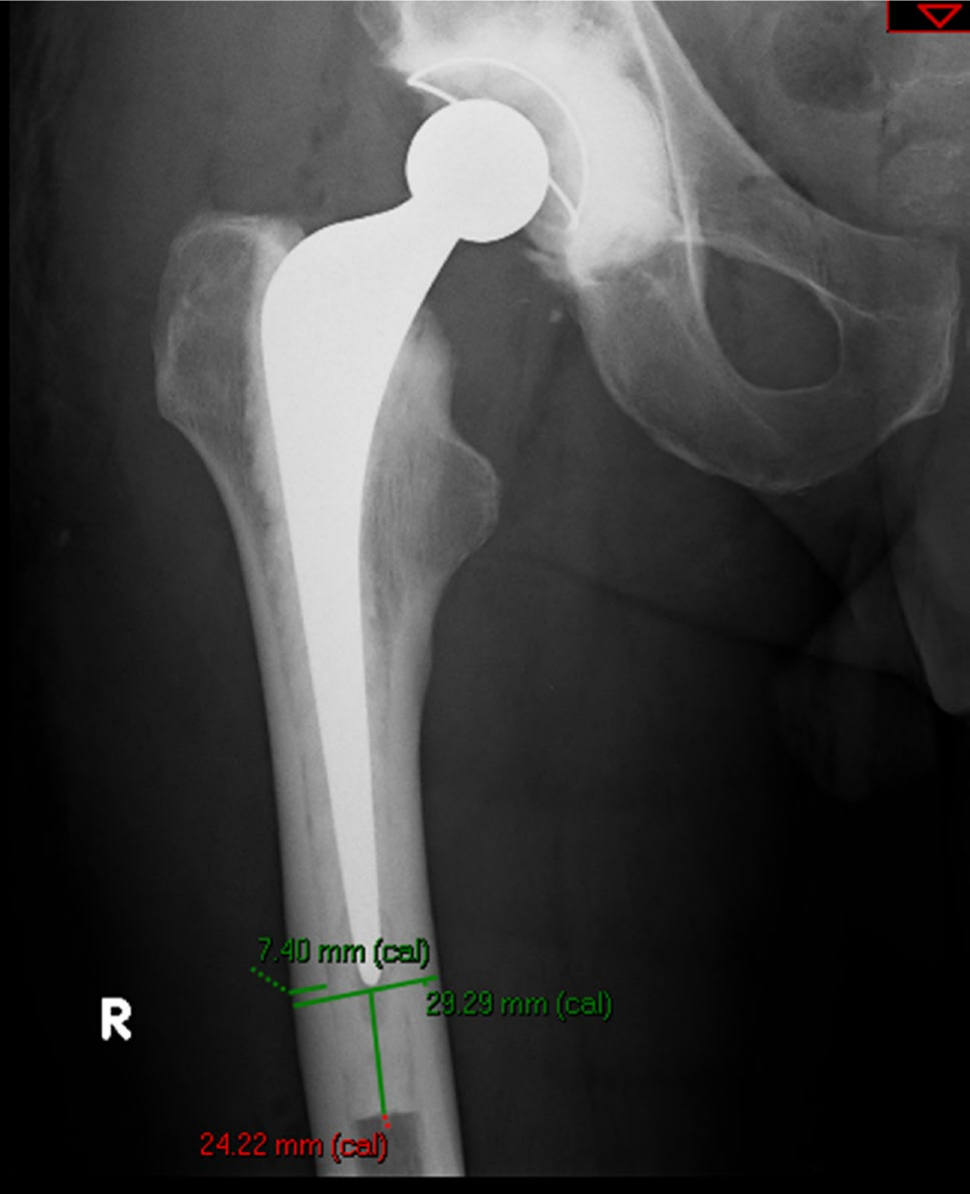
Radiographic measurements of cortical thickness, femoral diameter and length of distal cement mantle
